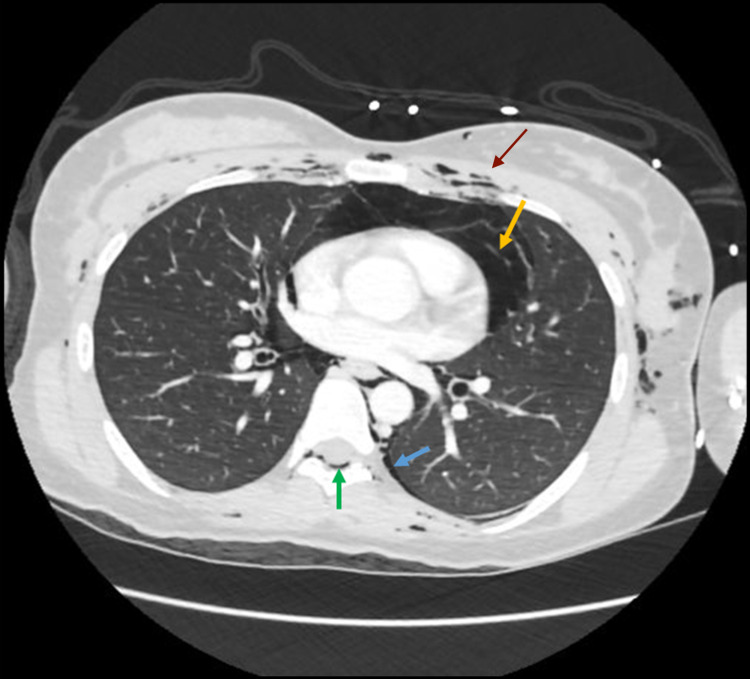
Computed tomography of the chest showing pneumothorax (blue arrow), pneumopericardium (yellow arrow), epidural pneumorrhachis (green arrow), and muscular emphysema (red arrow)
